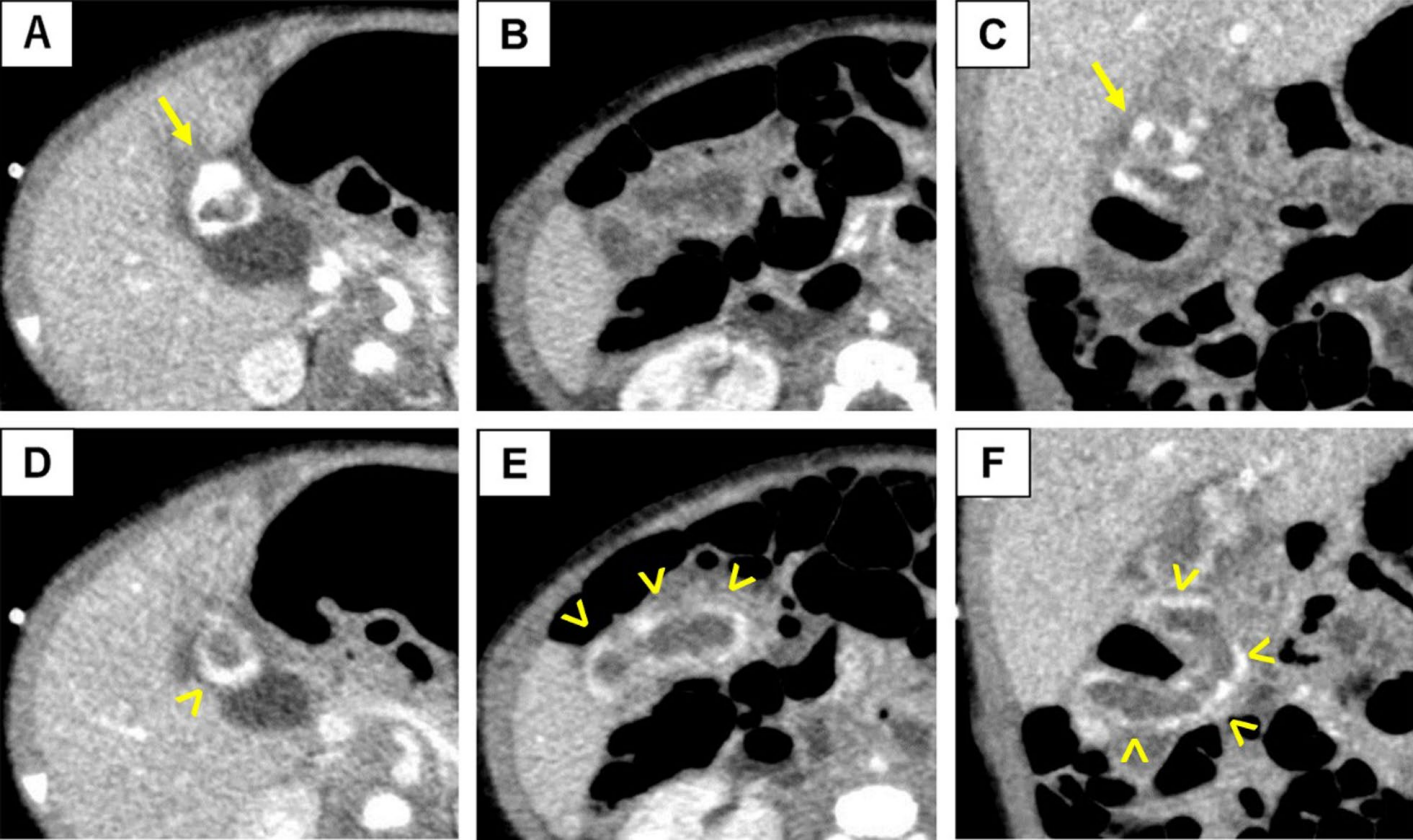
Abdominal contrast-enhanced computed tomography performed at 77 days of age. In the arterial phase (A and B on axial, C on sagittal), nodule-like lesions were strongly enhanced (arrow) near the portoenterostomy site. In the venous phase (D and E on axial, F on sagittal), enhanced lesions spread to the Roux limb (arrowhead). No dilated vessels and extravasation into the intestinal tract were observed
